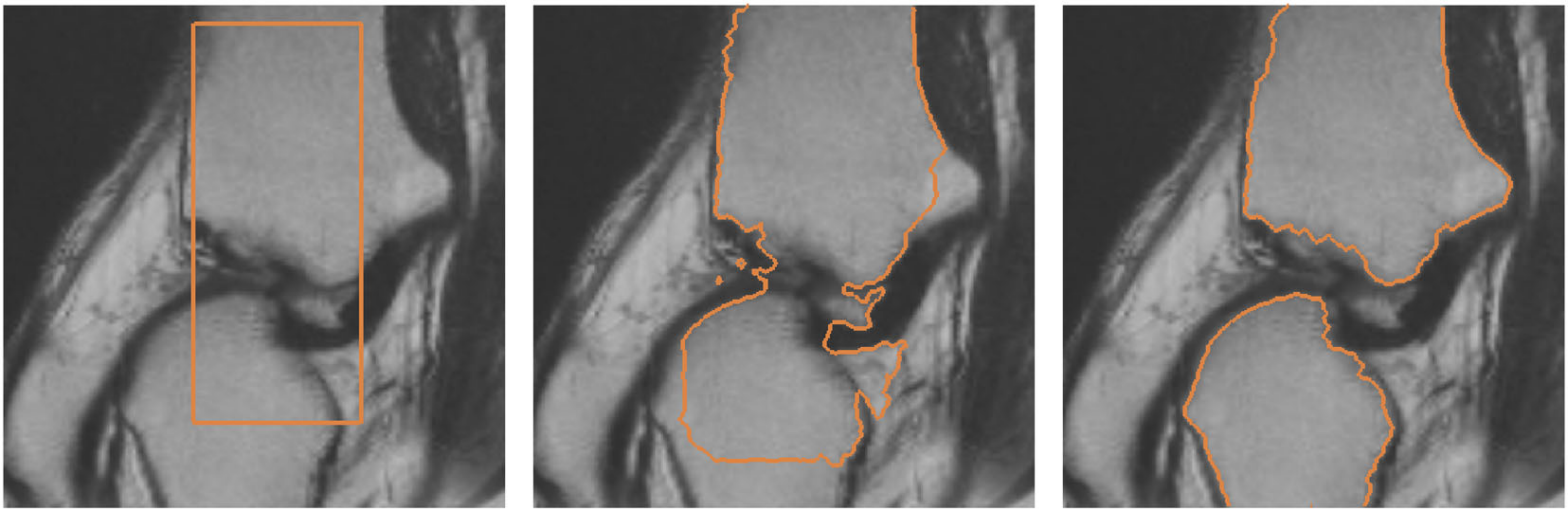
Segmentation of the knee from magnetic resonance image using gradient vector interaction-based and shape-based energies.
